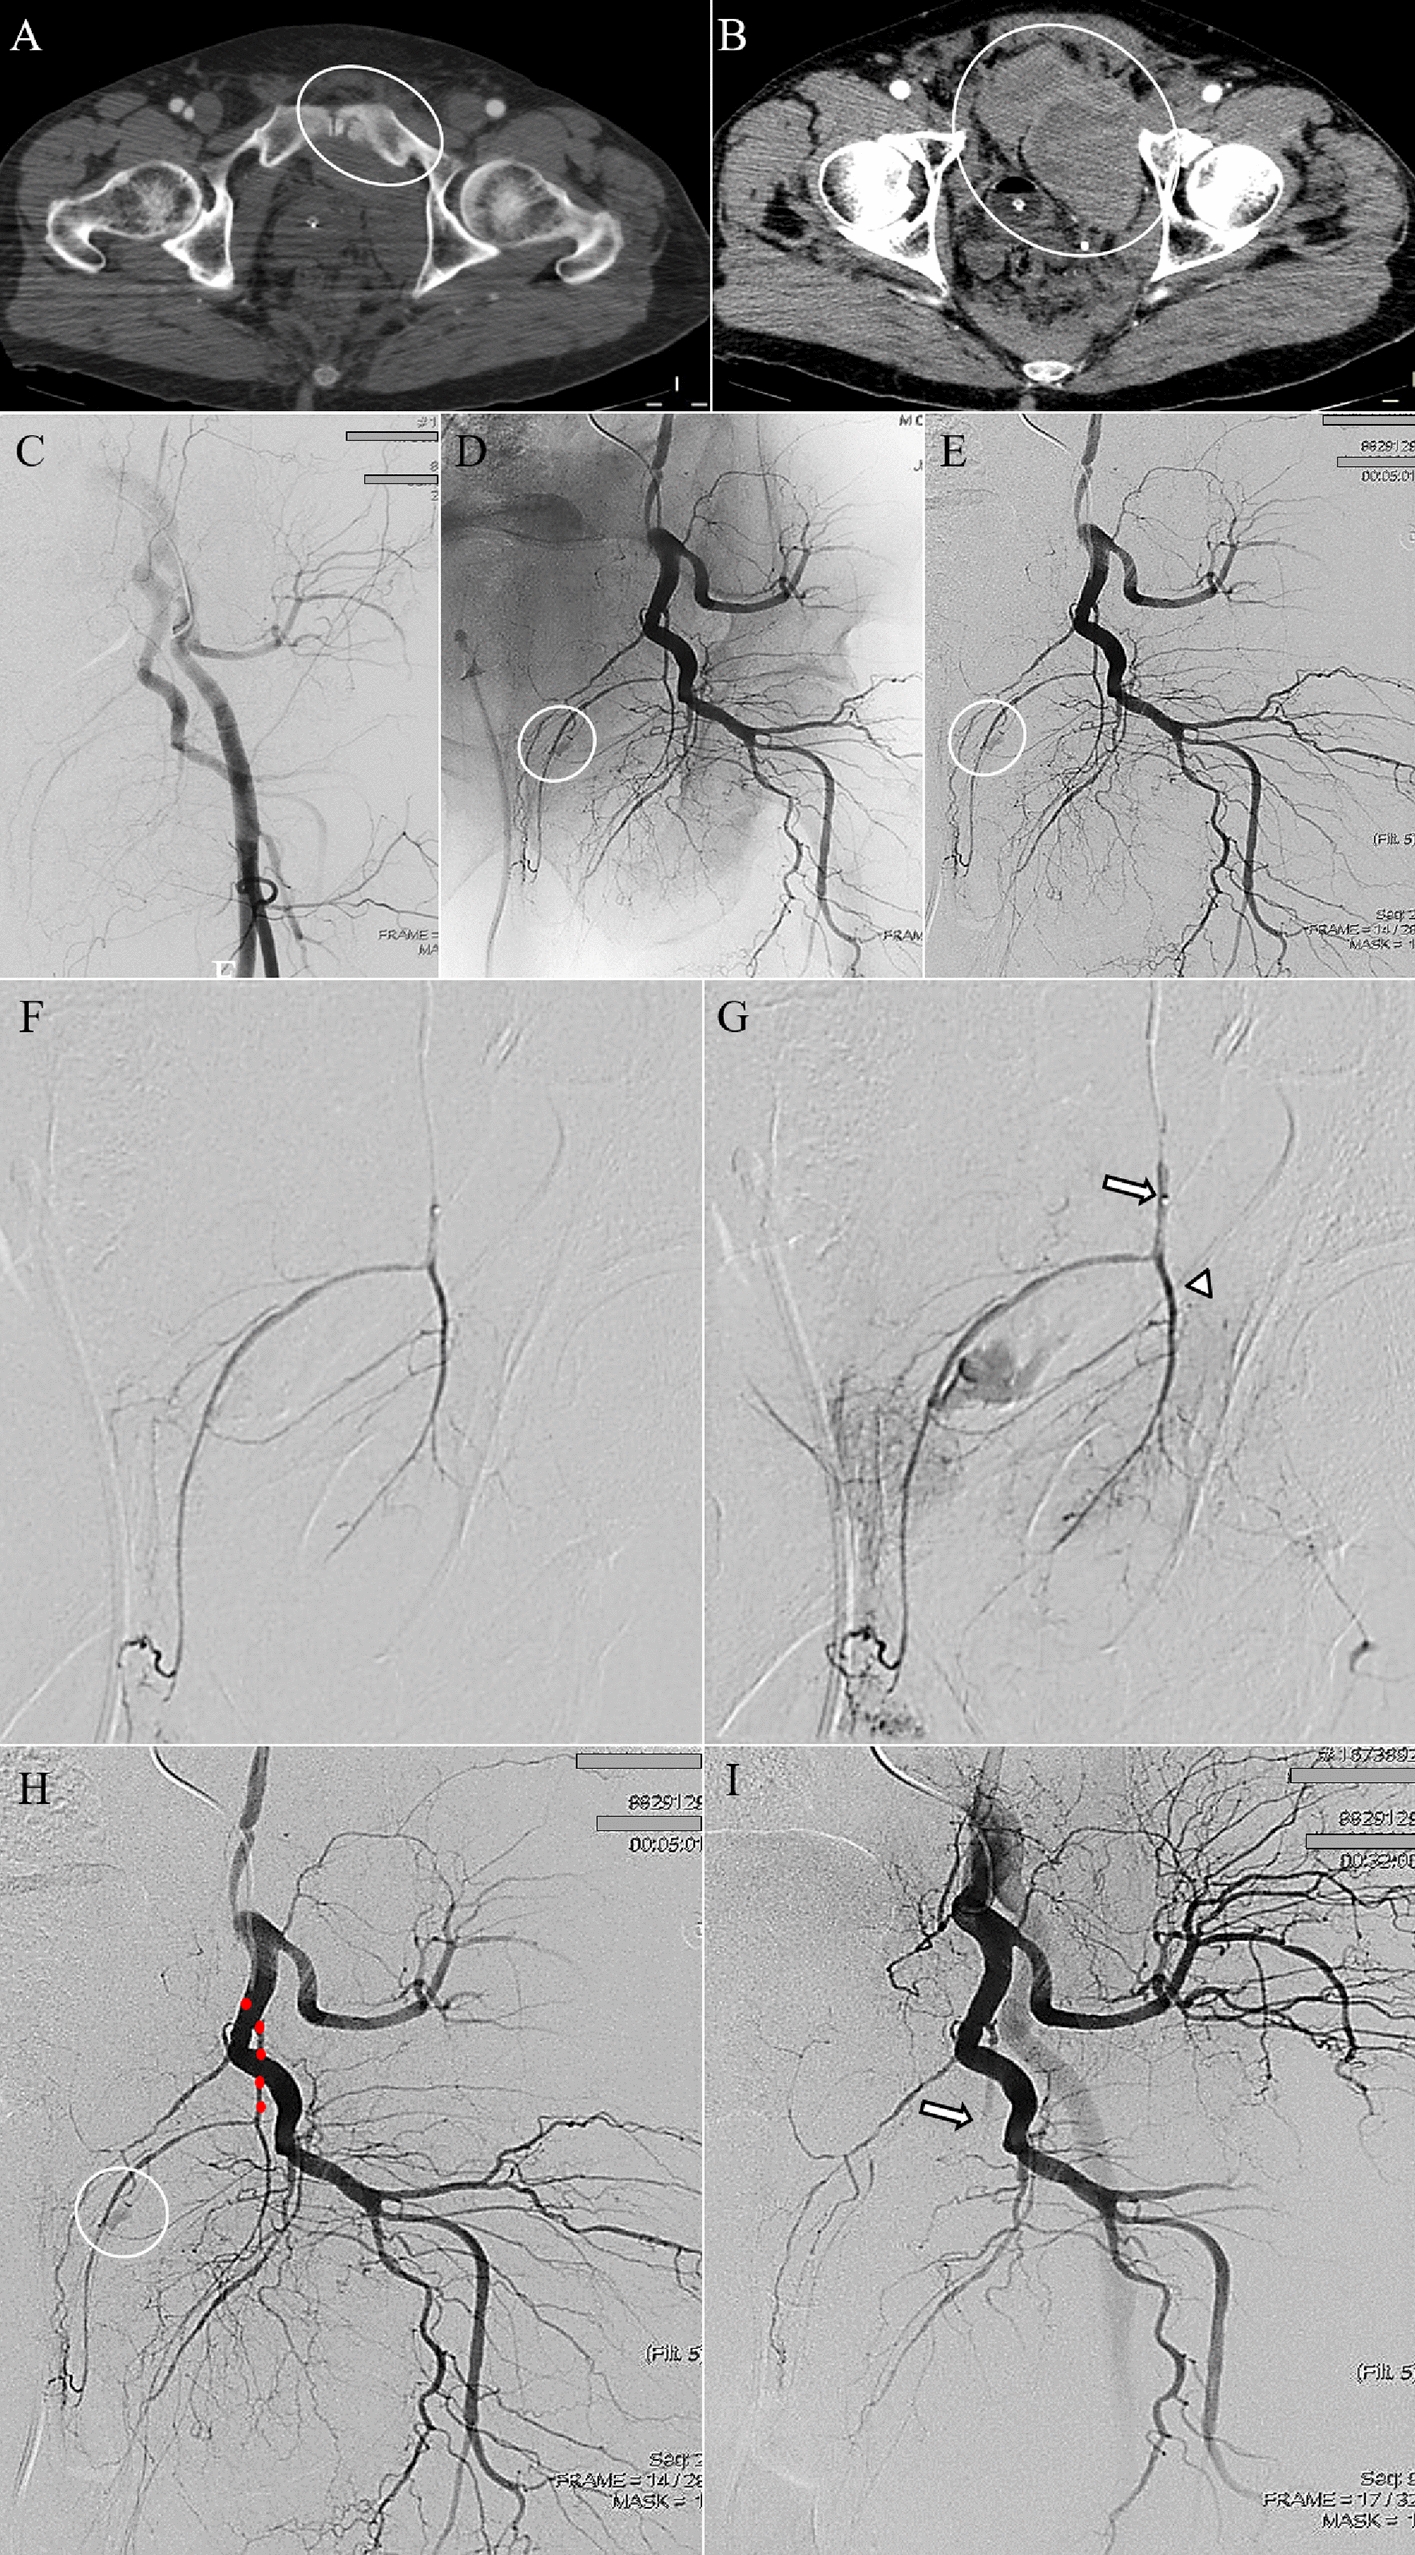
Axial CT of a stable patient with a fracture of the left pubic ramus (circled in A) and pelvic hematoma (circled in B). Proximal non-subtracted angiogram of the left external iliac artery (C) showing no focal “blush”. The subsequent selective study of the left internal obturator artery demonstrating the contrast blush [circled in subtracted image (D)] in correspondence consistent with injury lesions on CT and suitable for superselective embolization; digital subtracted image of the same patient, allowing a more accurate depiction of the arterial extravasation (circled in E). Selective catheterization of the injured artery (F). The arterial branch responsible for the blush was embolized with Glue, a definitive embolic agent (head arrow in G) while the upper obturator branch that supplies external genitalia is closed with Spongel, an absorbable embolic material (arrow in G). Compared to the pre-procedural angiography in which are highlighted the vessel (red points in H) responsible for bleeding (circled in H), the post-embolization angiogram confirms a successful occlusion only of the injured artery (arrow in I) with the regular patency of the remaining vessels
